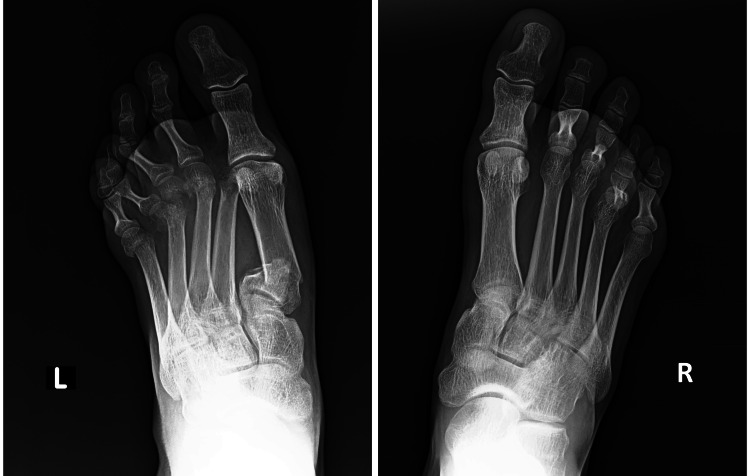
Anteroposterior X-ray view of the left and right foot after the accident Anteroposterior X-ray view of the left and right foot after the accident showing right foot third finger proximal phalanx open fracture, right foot fourth finger proximal phalanx fracture, left foot first metatarsal proximal fracture, left foot second, third, fourth, and fifth metatarsal displaced fractures, left foot cuboid, and navicular bone fractures. The patient's left foot's metatarsals were all fractured.
